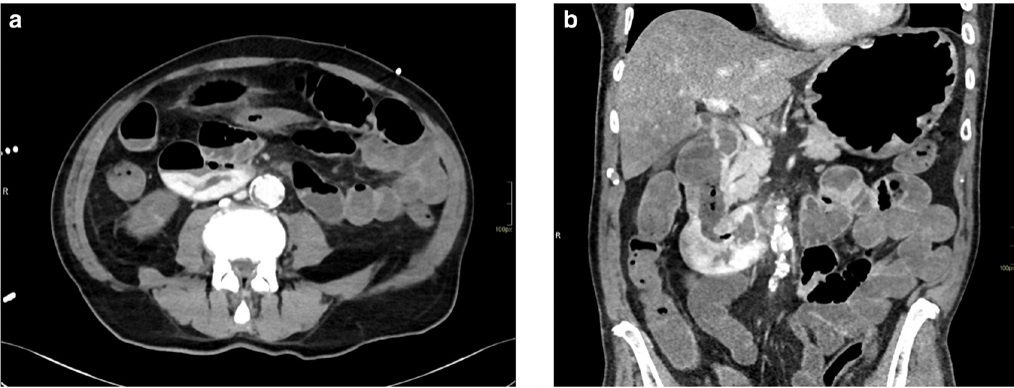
CT late phase of the study (respectively in axial and coronal plane) shows an active spreading of contrast medium in the third-fourth portion of duodenum.
